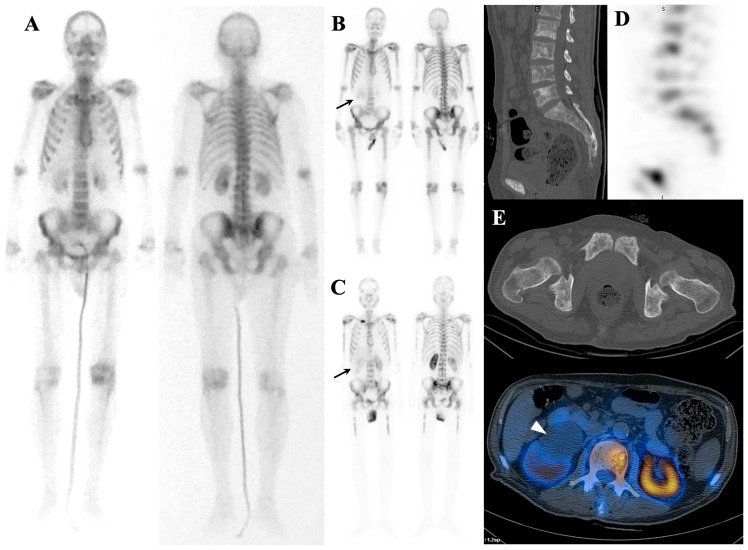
Atypical case of metastatic superscan in a 67-year-old man with biochemical persistence following radical prostatectomy (Gleason score 4 + 5 and initial PSA = 64 ng/mL). The patient was referred for ^99m^Tc-HYNIC-PSMA SPECT/CT and repeat bone scan due to highly elevated PSA levels, 3 months post-prostatectomy (PSA = 42 ng/mL). The initial bone scan was reported as negative (A), but a retrospective review revealed subtle, suspicious foci in the left lowest rib and right sacroiliac region. Subsequently, the recent bone scan (B) suggested a superscan, although the visualization of the kidneys was uncertain (black arrow). A few days later, PSMA imaging (C) was performed, unveiling widespread skeletal metastases (D) and indicating a poorly functioning right kidney and obstructive uropathy (black arrows in (B,C); white arrowhead in (E)). Notably, the right kidney showed more prominent abnormalities. It is important to note that non-visualization of the kidneys is not a prerequisite for a superscan and should be considered in prostate cancer patients with a long-standing history of benign prostatic hyperplasia, where kidney uptake may be preserved despite extensive bone metastases. This specific type of superscan is sometimes referred to as a sub-superscan or forme-frustré of a superscan.
